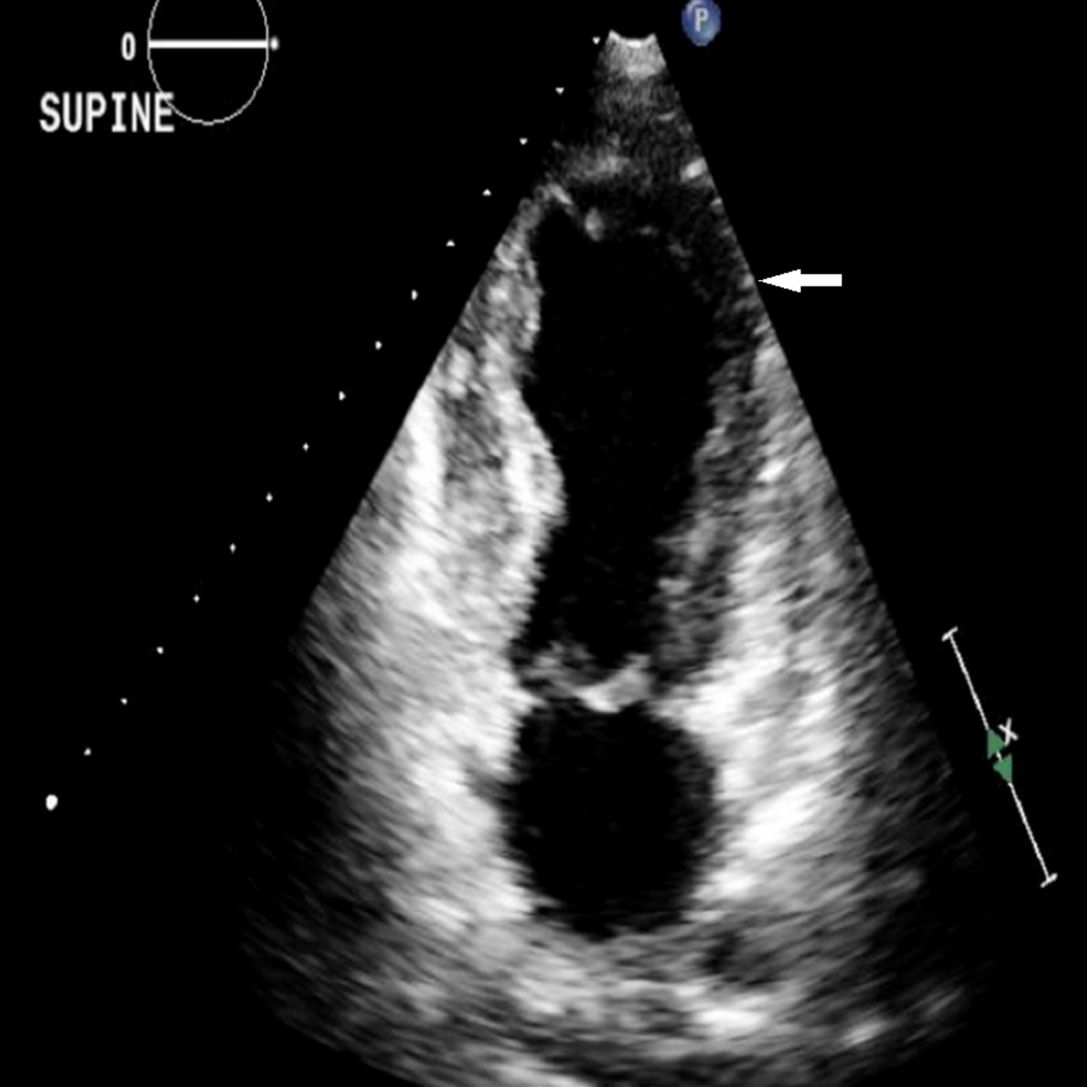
Two chamber end-systolic echocardiographic view showing apical ballooning (white arrow).
